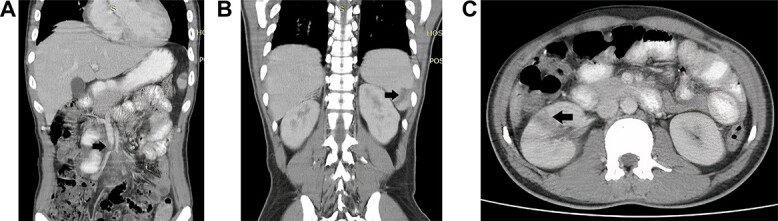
Computed tomography of the abdomen showing (a) thrombosis at the distal part of the superior mesenteric artery, (b) a wedge-shaped hypodense lesion in the lower pole of the spleen, (c) a hypodense lesion in the midpole of the right kidney.
